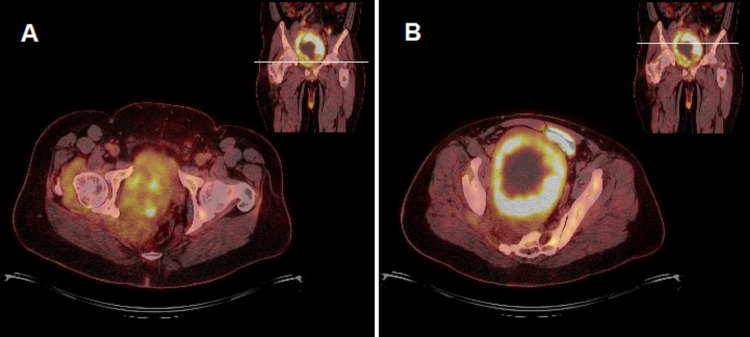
PET/CT axial images at the level of the hip (A) and the upper-middle portion of the pelvic mass (B). These images demonstrate areas of intense FDG avidity within the pelvic mass suggestive of malignant transformation. Much less avid and patchy FDG uptake of the neurofibroma can also be seen in the acetabulofemoral joint region.
